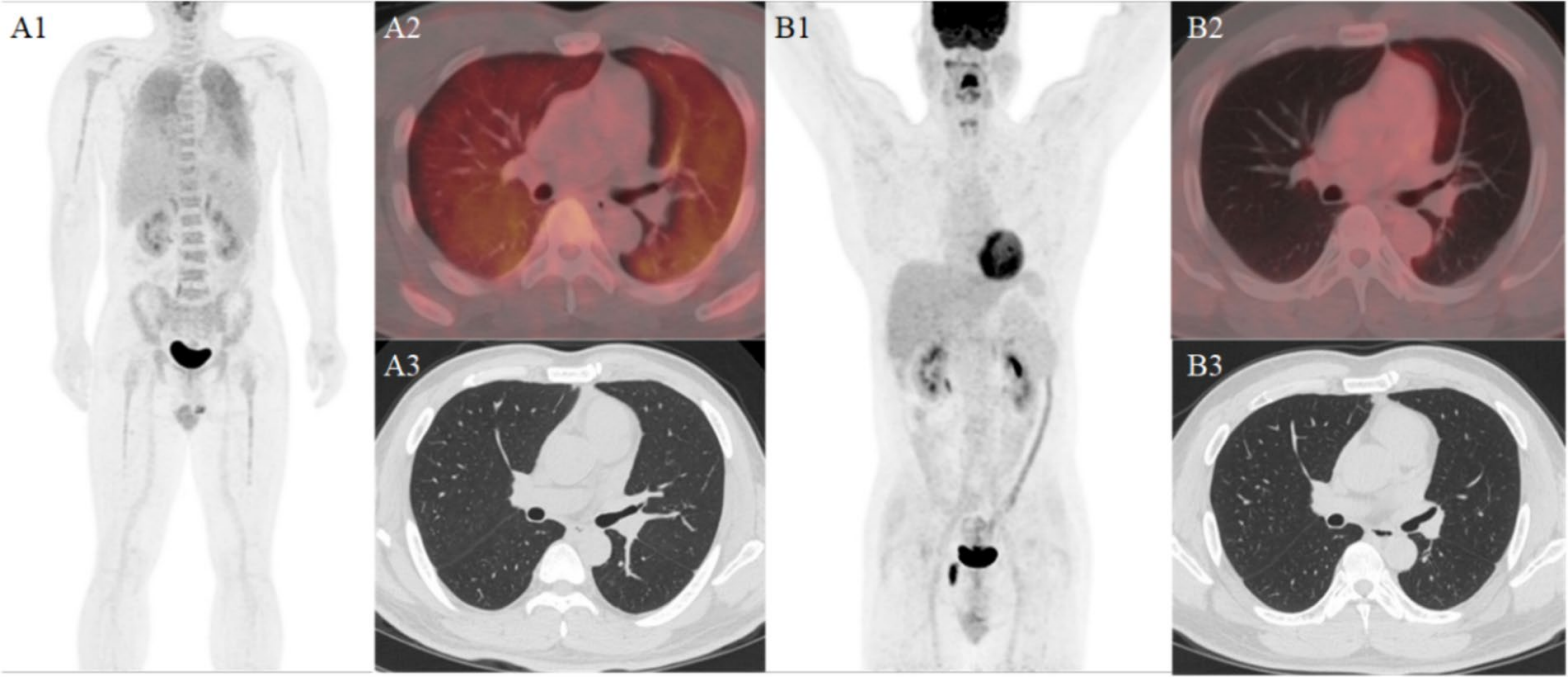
Panel (A1) shows diffuse increased metabolism in both lungs and the whole body bones. Panel (A2) shows diffuse increased metabolism in both lungs. Panel (A3) shows no obvious abnormalities in the high-resolution CT of the same machine. Panel (B1) shows no obvious abnormal metabolic increase in the whole body of the patient after treatment. Panel (B2) shows and no obvious abnormalities in the PET/CT fusion image. Panel (B3) shows and the high-resolution CT of the same machine.
